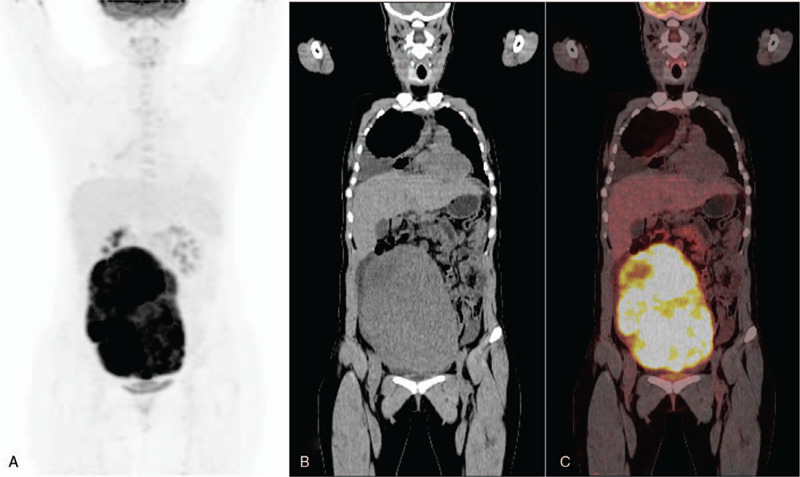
Preoperative PET/CT findings, Positron emission tomography (PET) with maximum grayscale projection (A). Coronal CT image showed a large mass in the abdominal cavity, with an uneven density, and a clear boundary (B). PET/CT fusion image showed uneven abnormal increase of tumor metabolism, with no abnormal hypermetabolic lesions in other sites (C).
